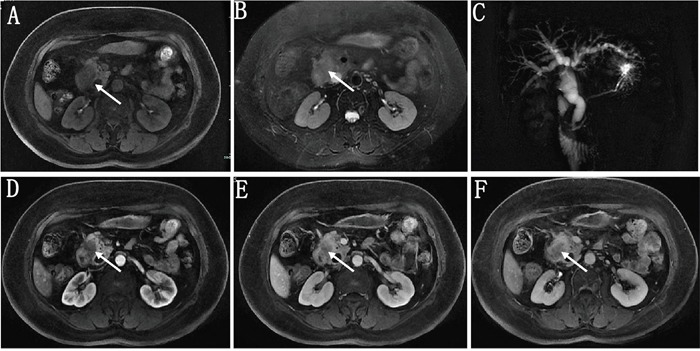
A 57 year old female patient with pathologically proven pancreatic ductal adenocarcinoma On fat-suppressed LAVA T1- (A) and T2- (B) weighted imaging, the tumor showed hypointensity and hyperintensity with an ill-defined boundary, respectively. MRI cholangiopancreatography (MRCP) showed the common bile duct and intra-, extrahepatic bile ducts were markedly dilated (C). Gadolinium enhanced images in arterial (D), portal (E) and delayed phase (F) both showed the tumor were hypointensity compared with the pancreatic parenchyma.
